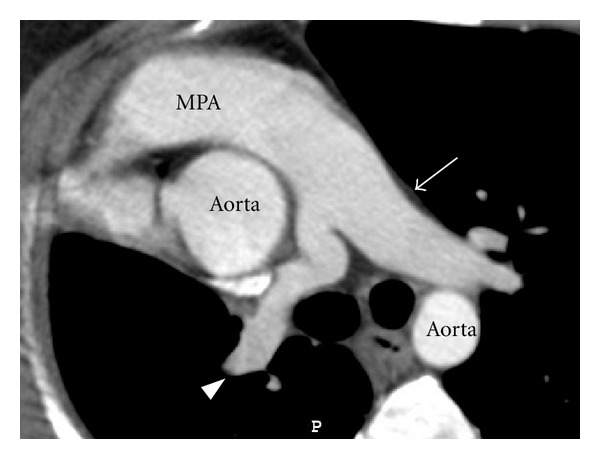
CT angiography axial image depicts contour abnormality of right pulmonary artery (arrow head), Normal caliber left pulmonary artery (thin arrow), Main pulmonary artery (MPA).
